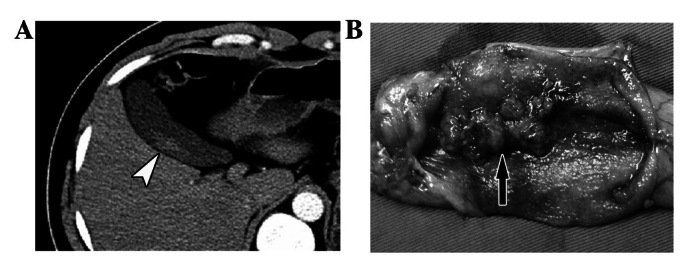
Tubular adenoma from a 28-year-old male. (A) Computed tomography (CT) image shows a lesion parallel with the wall of the gallbladder. There is space between the mass and wall and clear space between the gallbladder and the liver (white arrowhead). (B) Gallbladder removed by laparoscopy (black arrow, narrow polyp with a tiny base).
